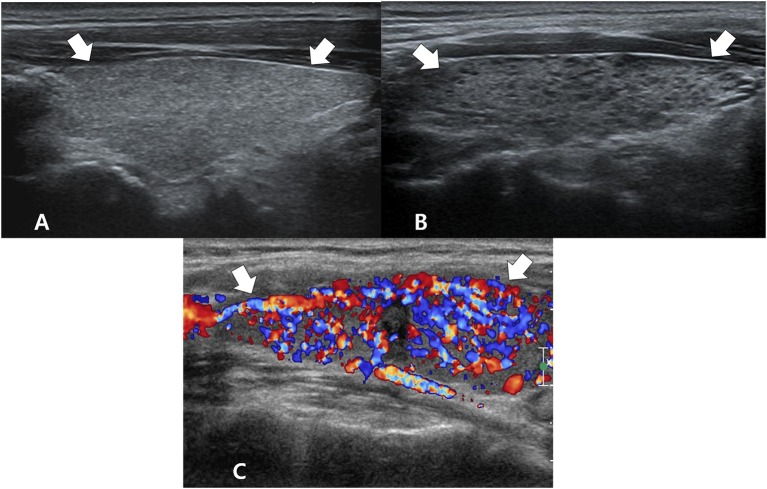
Ultrasonography images of essential and specific features for diffuse thyroid disease: coarse echotexture (A) and micronodulation (B) on longitudinal gray-scale sonograms and thyroid inferno (with poor delineation of thyroid parenchyma due to diffuse color signals) (C) on a longitudinal color Doppler sonogram. The arrows mark the thyroid gland.
